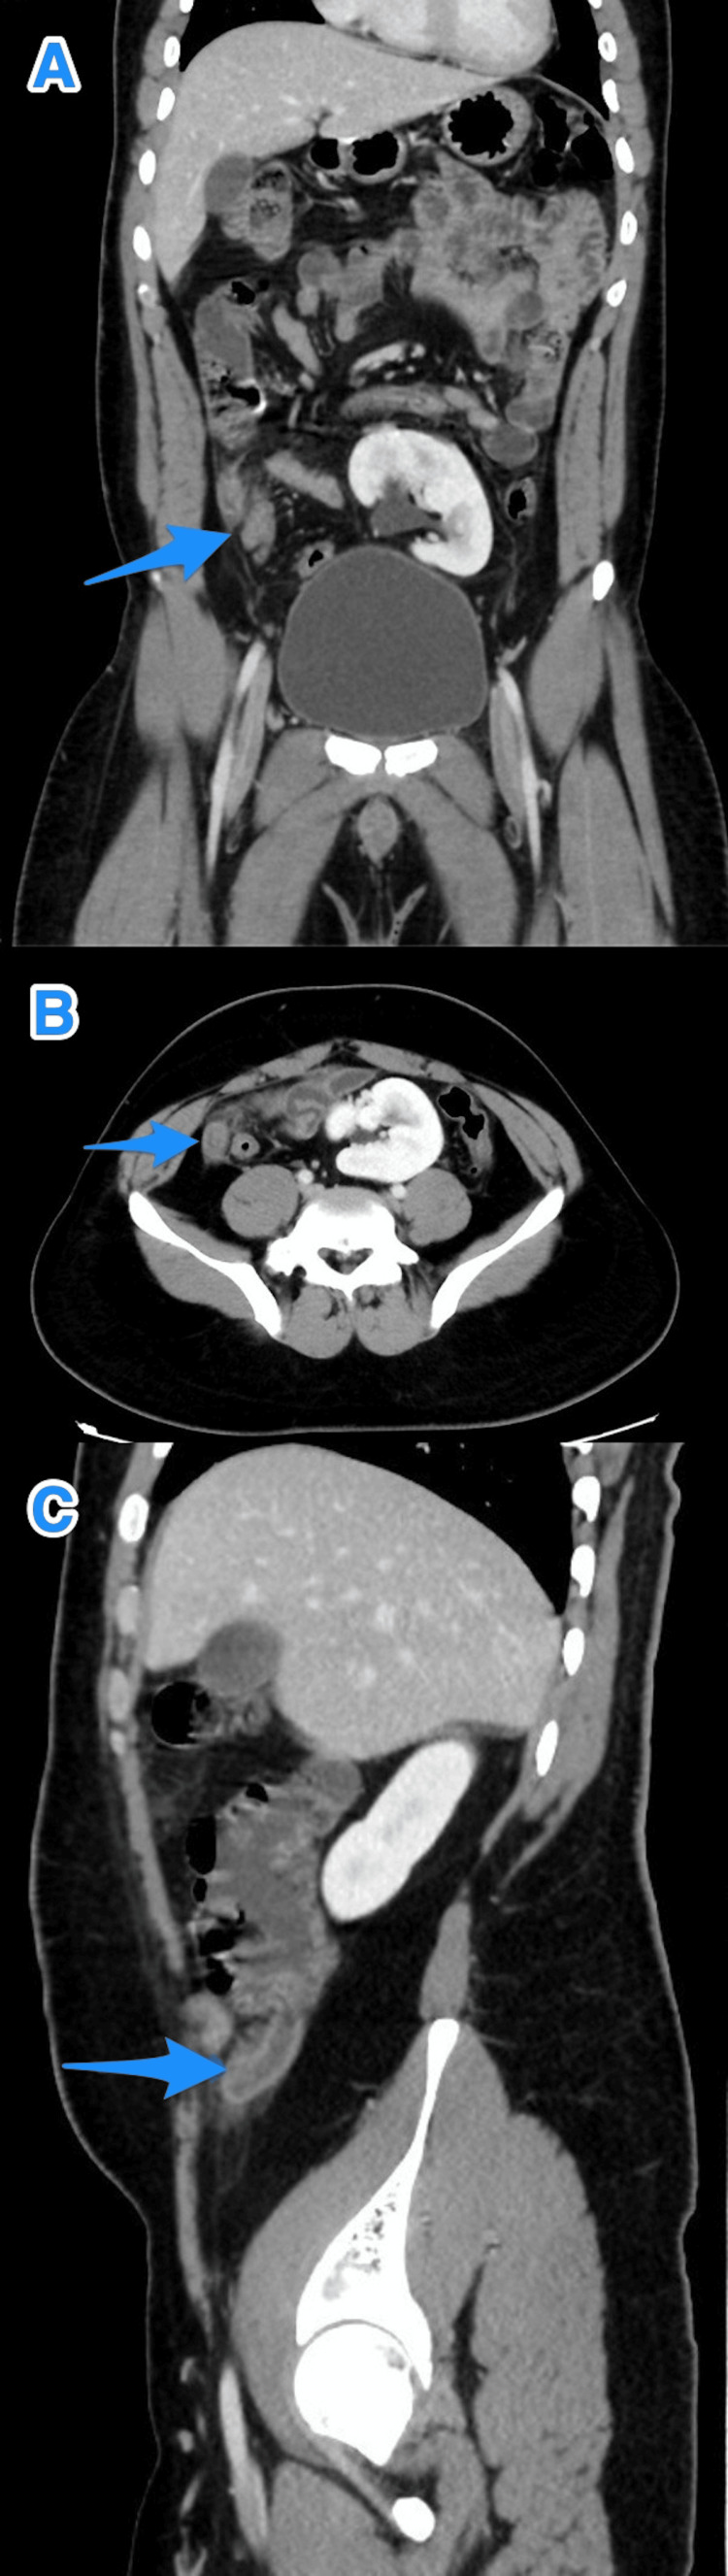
A multiview computed tomography abdomen scan (A-C) shows a small diverticulum close to the tip of an inflamed appendix (arrows).
